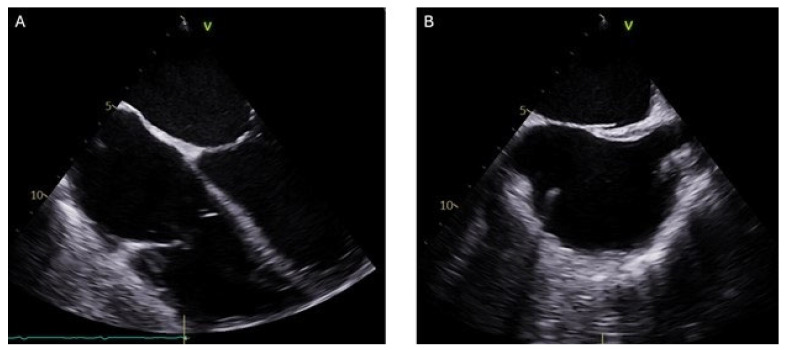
Post-Procedure transesophageal echocardiogram: (A) 2D 0° 4-chamber RV-focused view with no residual thrombus. (B) 2D 101° bi-caval view confirming absence of thrombus and preserved caval inflow.
